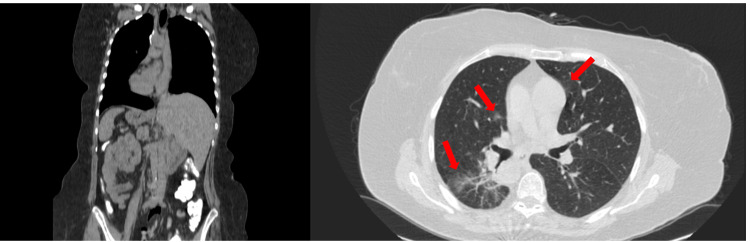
Coronal (left side) and axial views (right side) of computed tomography of the abdomen and thorax. Ground glass infiltrates (arrows) in the right lower and middle pulmonary lobe and the left upper pulmonary lobe; findings suggestive of early stages of COVID-19 infection. COVID-19: coronavirus disease 2019
